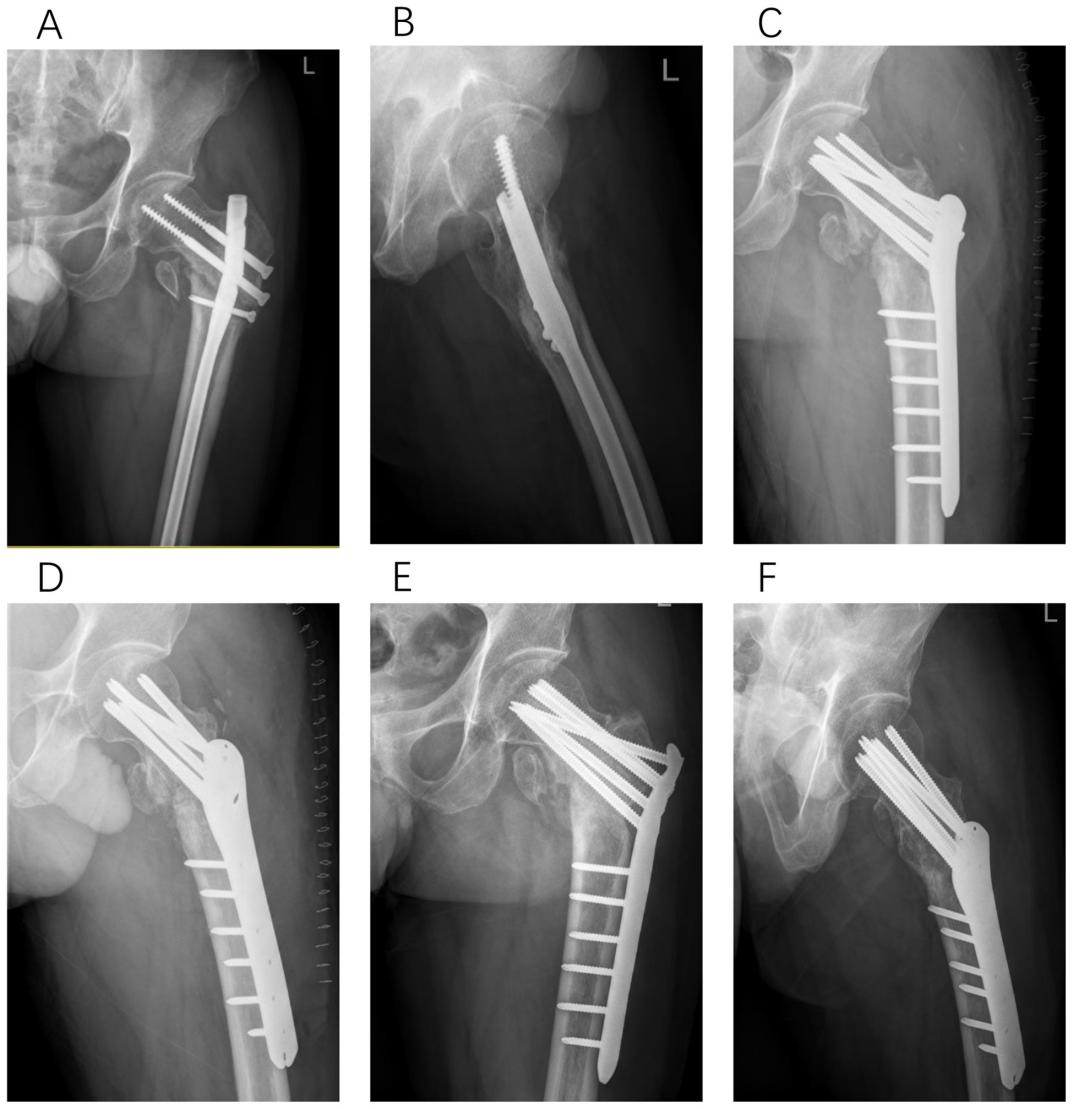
Type II-A, a 51-year-old male patient underwent femoral interlocking nail fixation for a left intertrochanteric femoral fracture. More than 12 months post-operatively, radiographic evaluation revealed the intramedullary nail was broken. In the subsequent revision surgery, we removed the broken nail, corrected the varus deformity via osteotomy, and implemented plate internal fixation. Successful bony union was achieved 3 months following this revision. (A, B) Anteroposterior and lateral X-rays of the femur obtained 12 months after initial femoral interlocking nail fixation. (C, D) Anteroposterior and lateral X-rays of the femur immediately following the revision surgery. (E, F) Successful bony union confirmed 3 months post-revision surgery.
